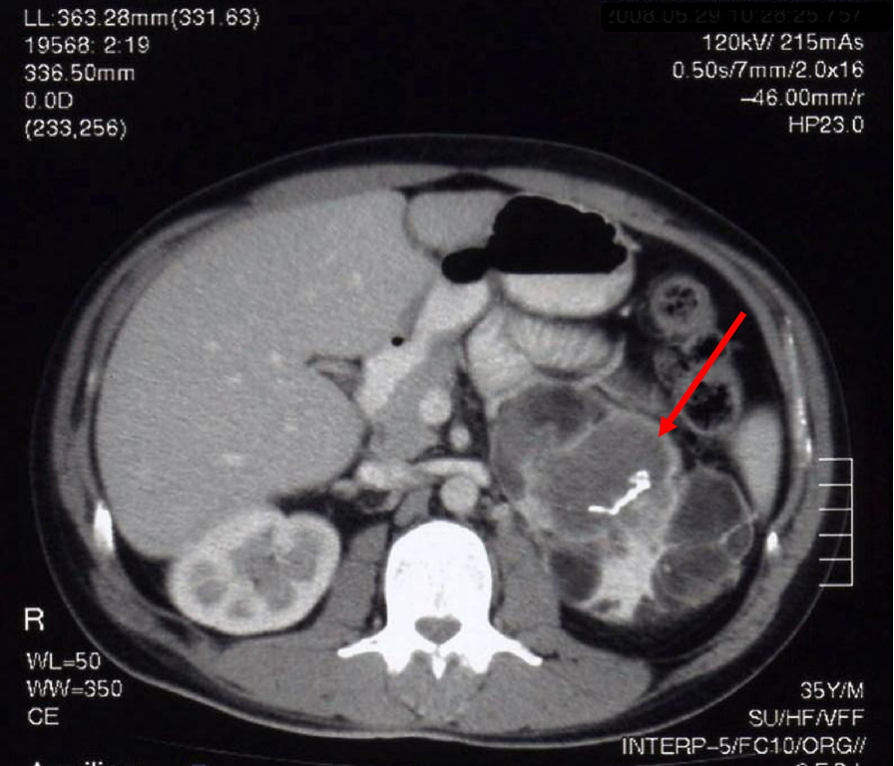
Abdominal computed tomography scan. Abdominal computed tomography scan before surgery showing the very enlarged and heterogeneous left adrenal gland (*arrow*) with soft tissue, fat and calcium attenuation.
